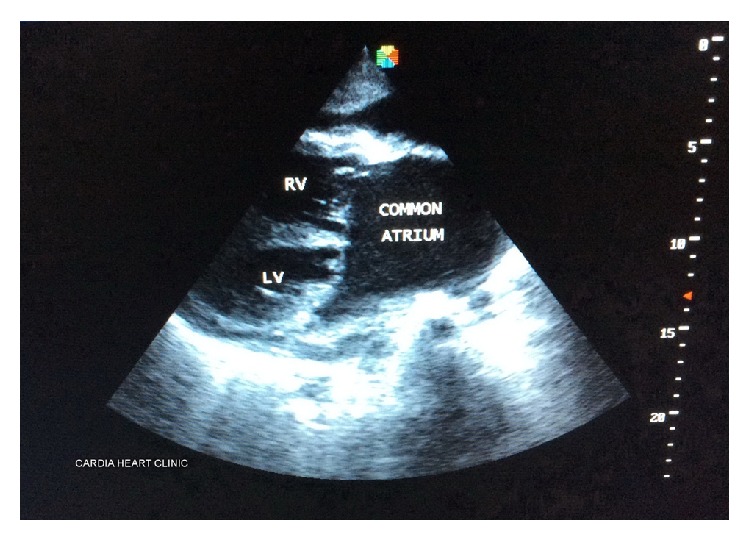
Two-dimensional echocardiogram parasternal long-axis view of the common atrium.
